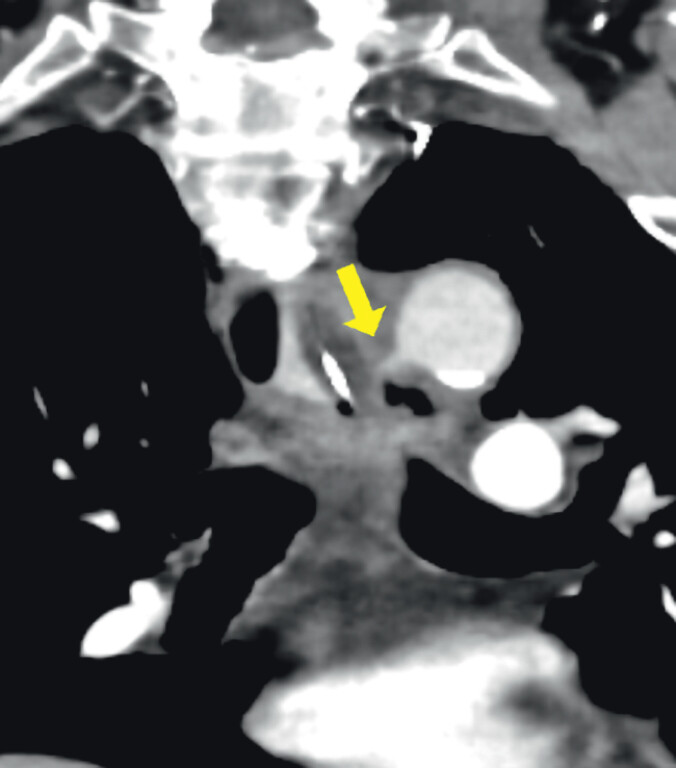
Contrast-enhanced computed tomography showing an aorto-esophageal fistula at the site of the tumor.
